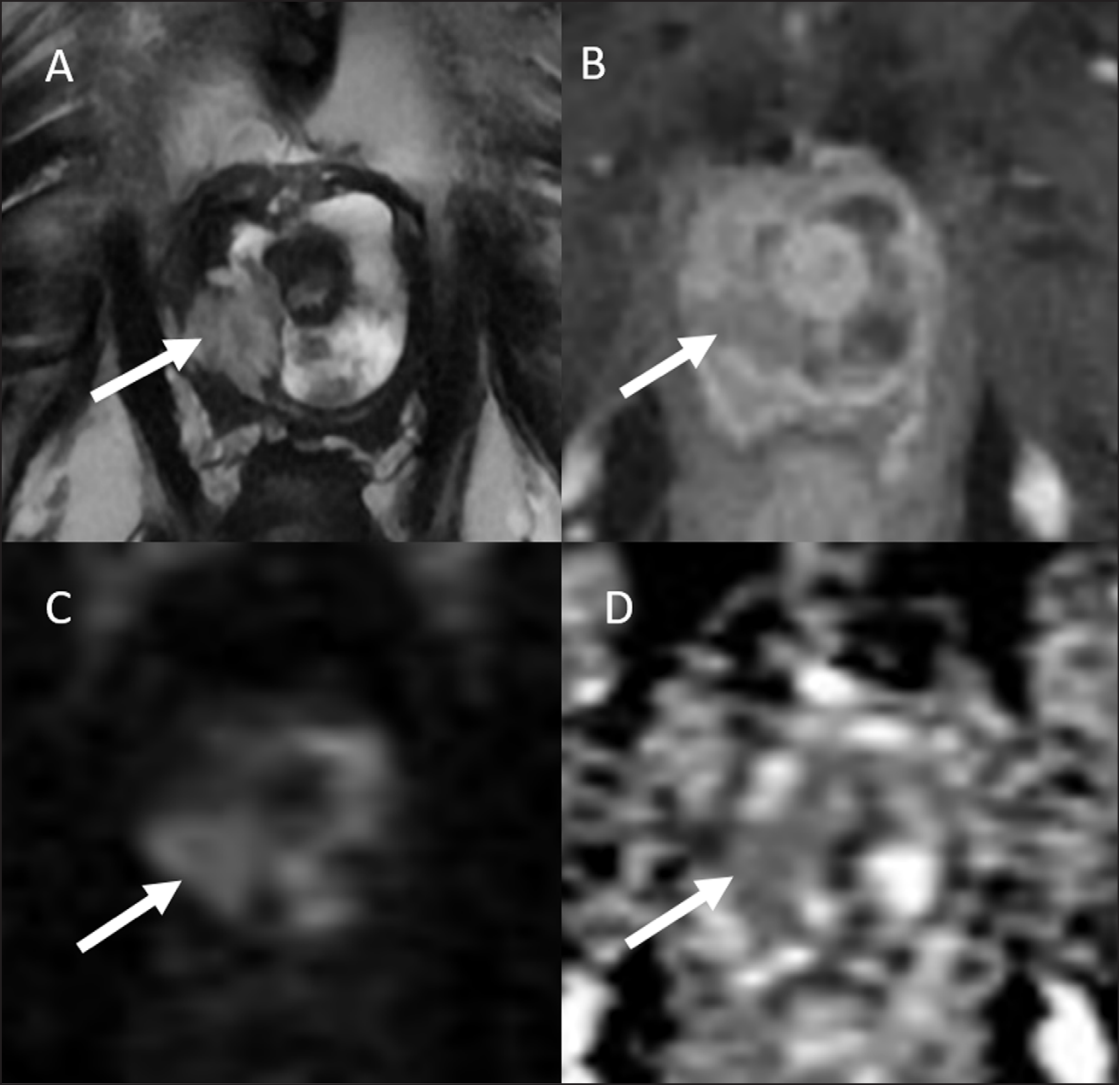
The enhancement of the intra-diverticular neoplasm is also well seen on sagittal T1-weighted fat-suppressed images (Figure 3) with a significant enhancement difference between the malignant portion (Figure 3A, white arrow) and the cystic portion (Figure 3B, white arrow) of the urethral diverticulum (Figure 3B, white arrow).
